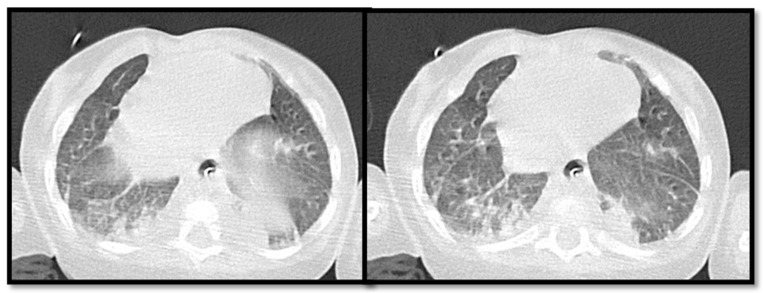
Pulmonary high-resolution CT scan (HRCT) performed after 14 days of the beginning of trametinib therapy. There are multiple parenchymal lung consolidations associated with diffuse ground glass opacities.
